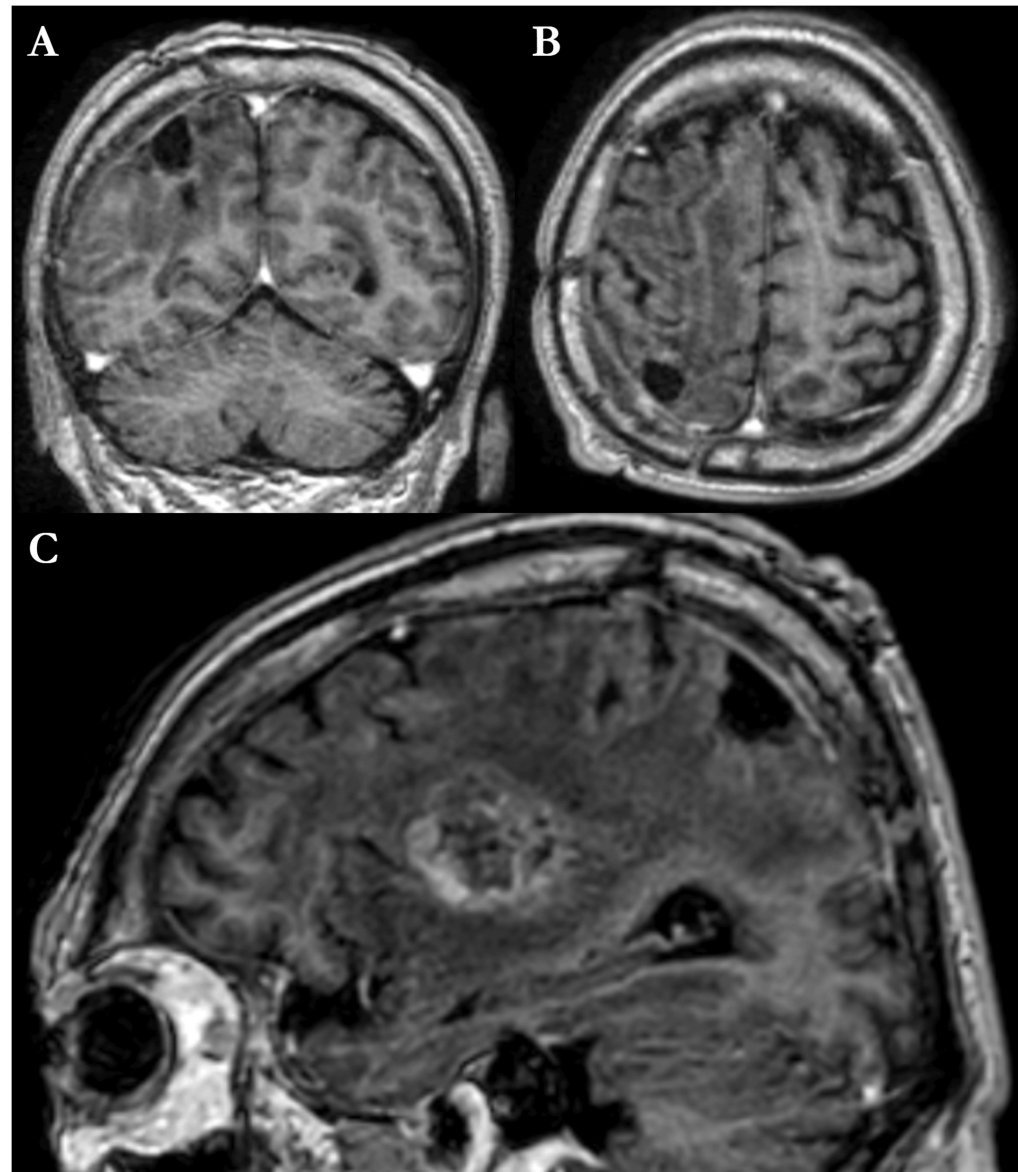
Early postoperative contrast-enhanced brain MRI (A) Frontal T1-weighted contrast-enhanced sequence. (B) Axial T1-weighted contrast-enhanced sequence. (C) Right paramedian sagittal T1-weighted contrast-enhanced sequence. Early postoperative contrast-enhanced brain MRI demonstrated the complete removal of the right parietal lesion, while the right insular lesion exhibited a volumetric increase.
